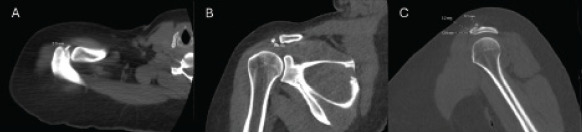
Computed tomography imaging without intravenous contrast of the right shoulder demonstrating A) axial, B) coronal, and C) sagittal series. A 13×3×5 millimeter calcific deposit overlying the anterior aspect of the acromioclavicular joint adjacent to the acromion consistent with hydroxyapatite deposition disease (dotted lines) is seen. No fracture or dislocation was visualized.
